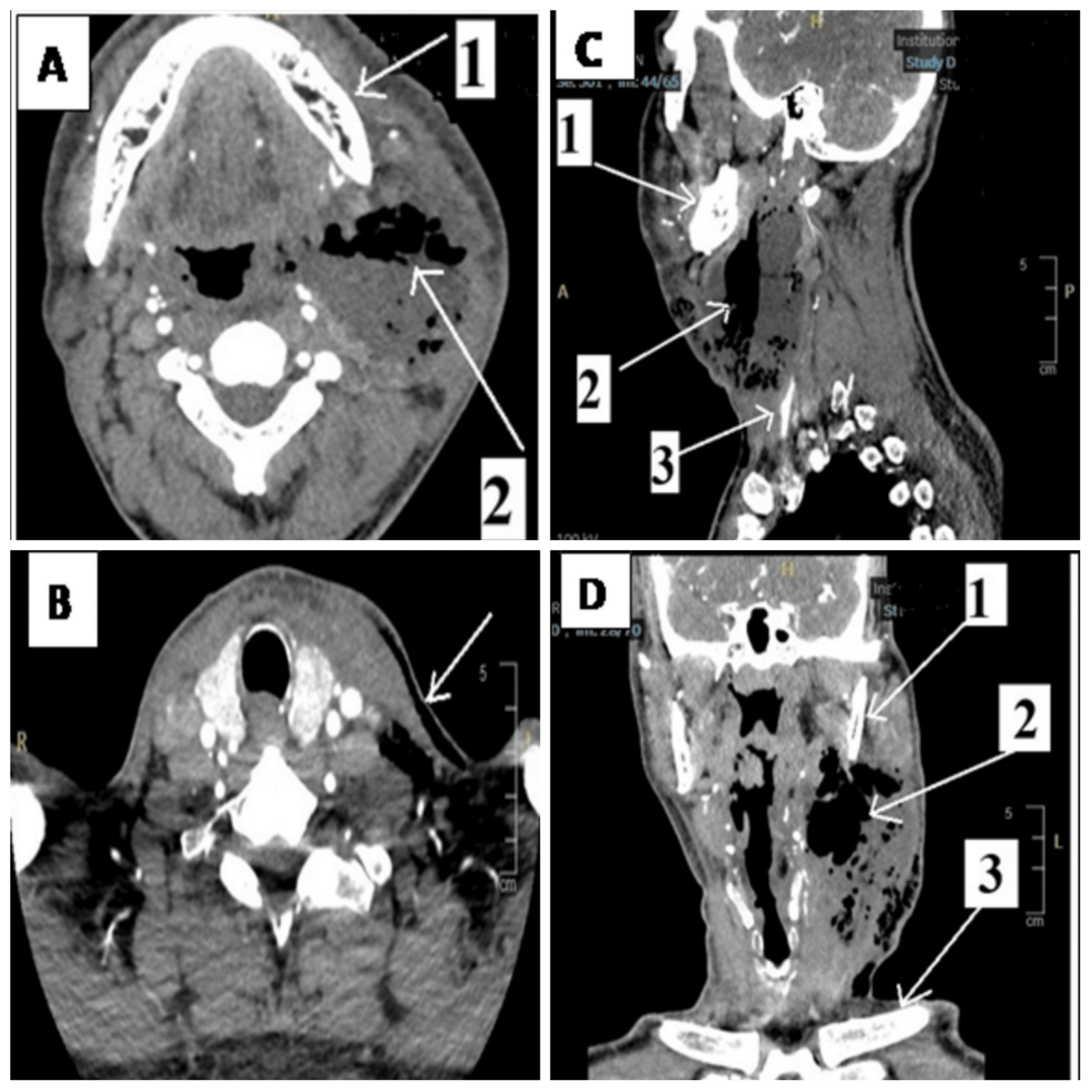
CT images showing the extent of necrotizing infection. (A) Axial view of the upper boundary: arrow 1 (mandible), arrow 2 (air and fluid). (B) Axial view of the lower boundary: arrow (subcutaneous air). (C) Sagittal and (D) coronal views: arrow 1 (mandible), arrow 2 (fluid with air shadow), and arrow 3 (clavicle).
